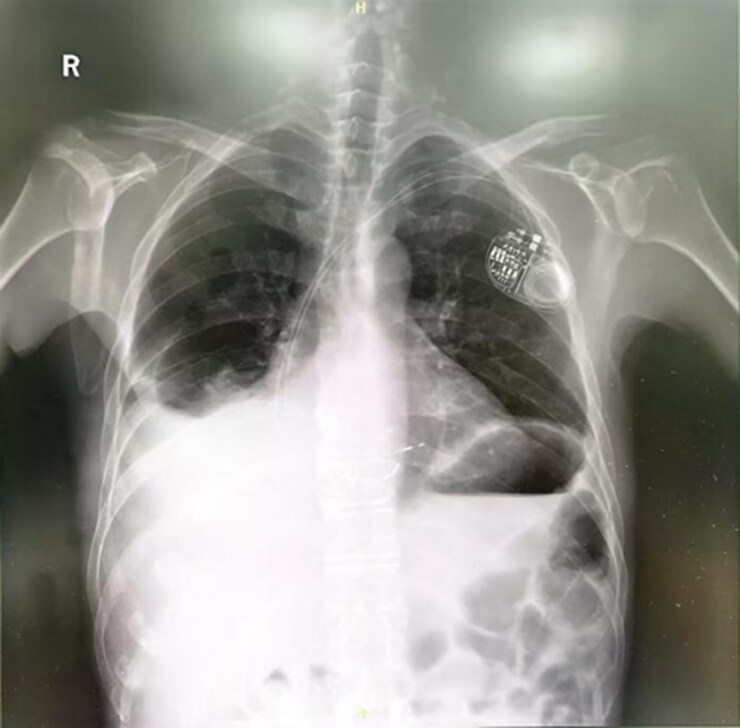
Chest X-ray after pacemaker implantation. The electrode leads were positioned in the right atrial appendage and the interventricular septum.
